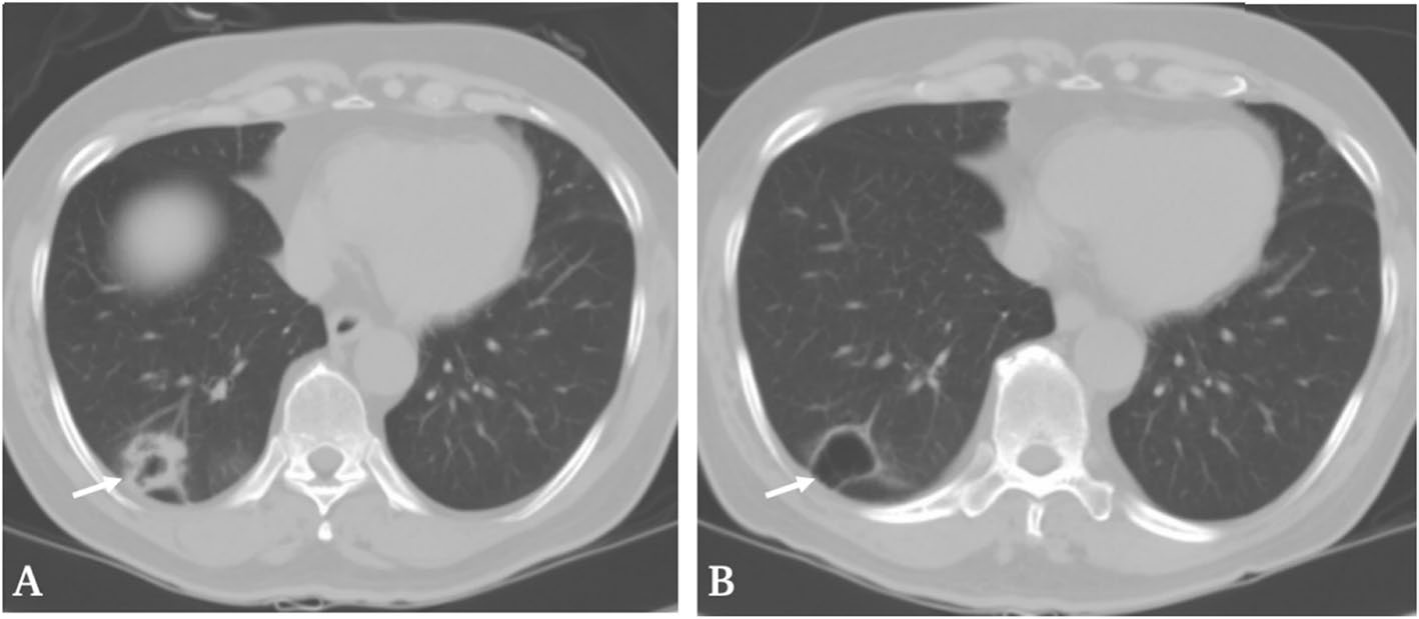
Chest computed tomography (CT) scans. A chest CT performed in June 2020 showing a 2.5 cm tumor in the low lobe of the right lung (white arrow). B chest CT performed in August 2020 showing a slight reduction in the diameter (2.4 cm) and marked reduction of solid component of the lung tumor (white arrow)
